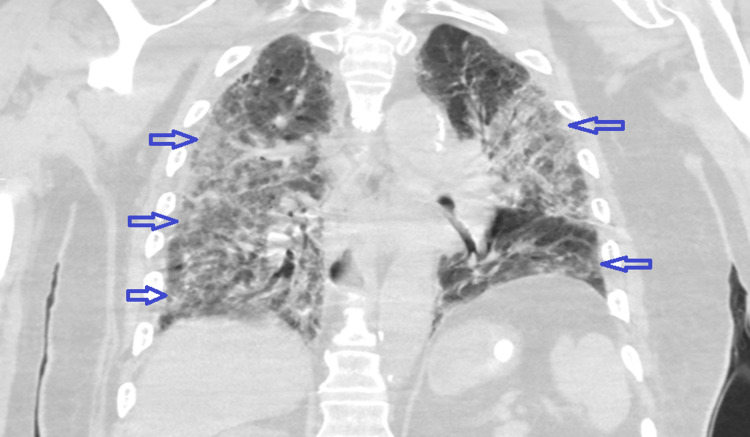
CT chest without contrast (coronal view) demonstrating diffuse bilateral pulmonary opacities (blue arrows).
